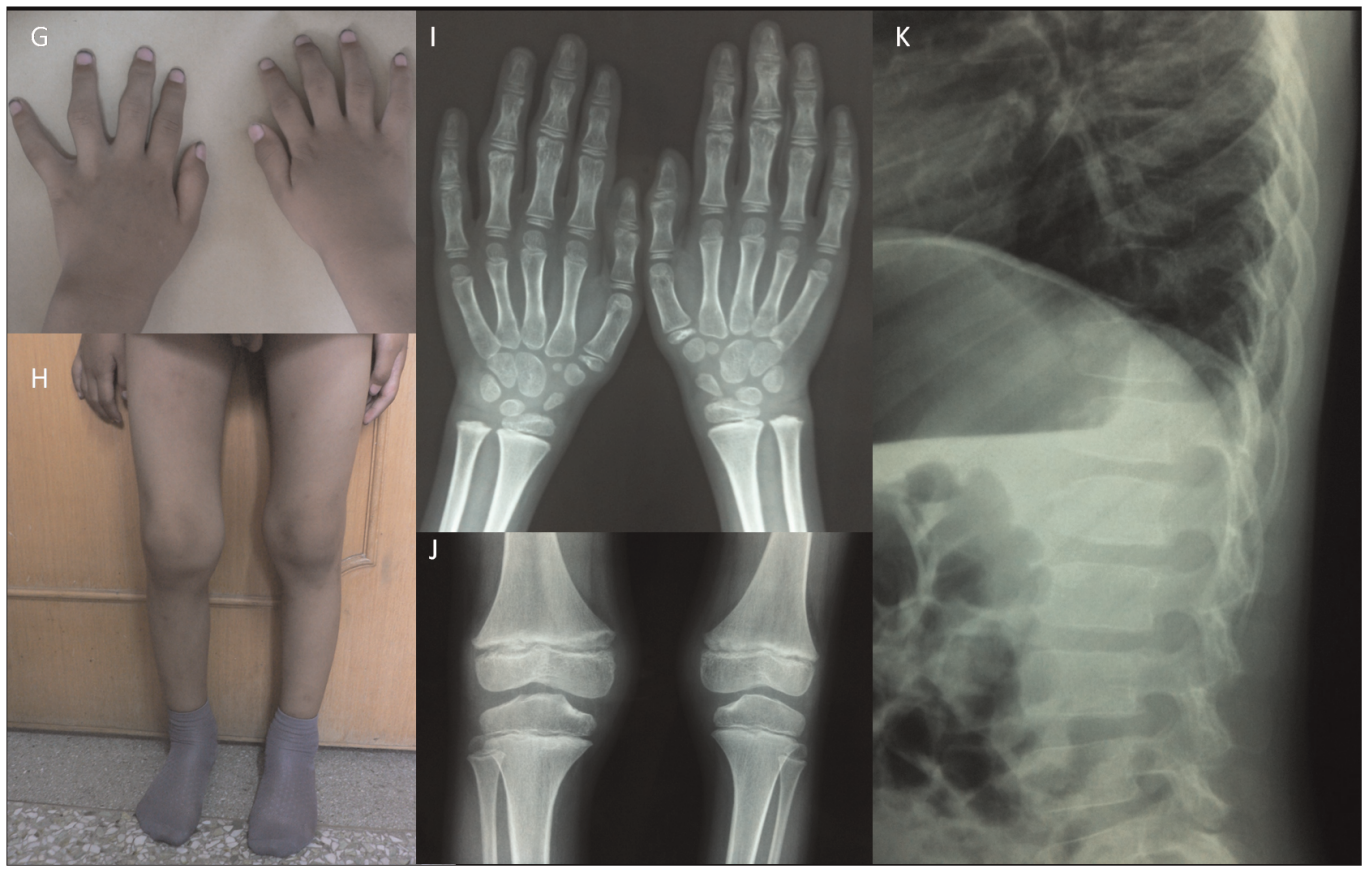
The photographs and X-ray images of patient II 2 in family 2. (G) The hands display interphalangeal joint swelling; (H) a swollen knee; (I) slight enlargement of the metacarpophalangeal epiphyses; (J) slight enlargement of the femoral and tibial epiphyses; (K) an increased anteroposterior diameter of the vertebral bodies and irregular vertebral end-plates.
